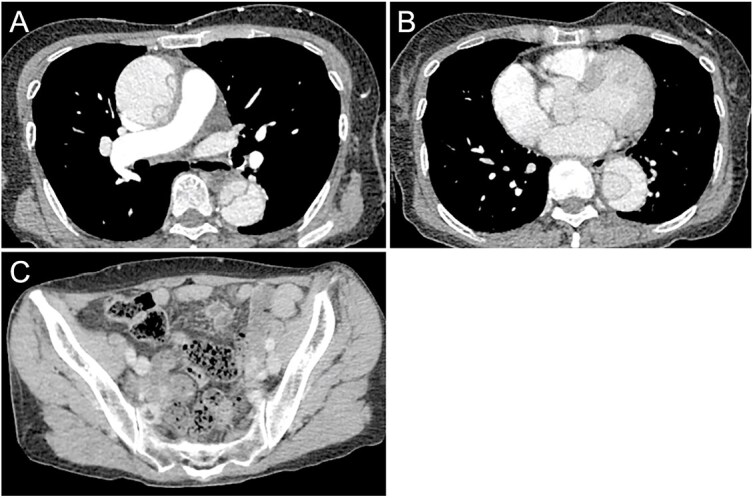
Contrast-enhanced CT showing type A acute aortic dissection. No thrombus was observed in the iliac veins.
